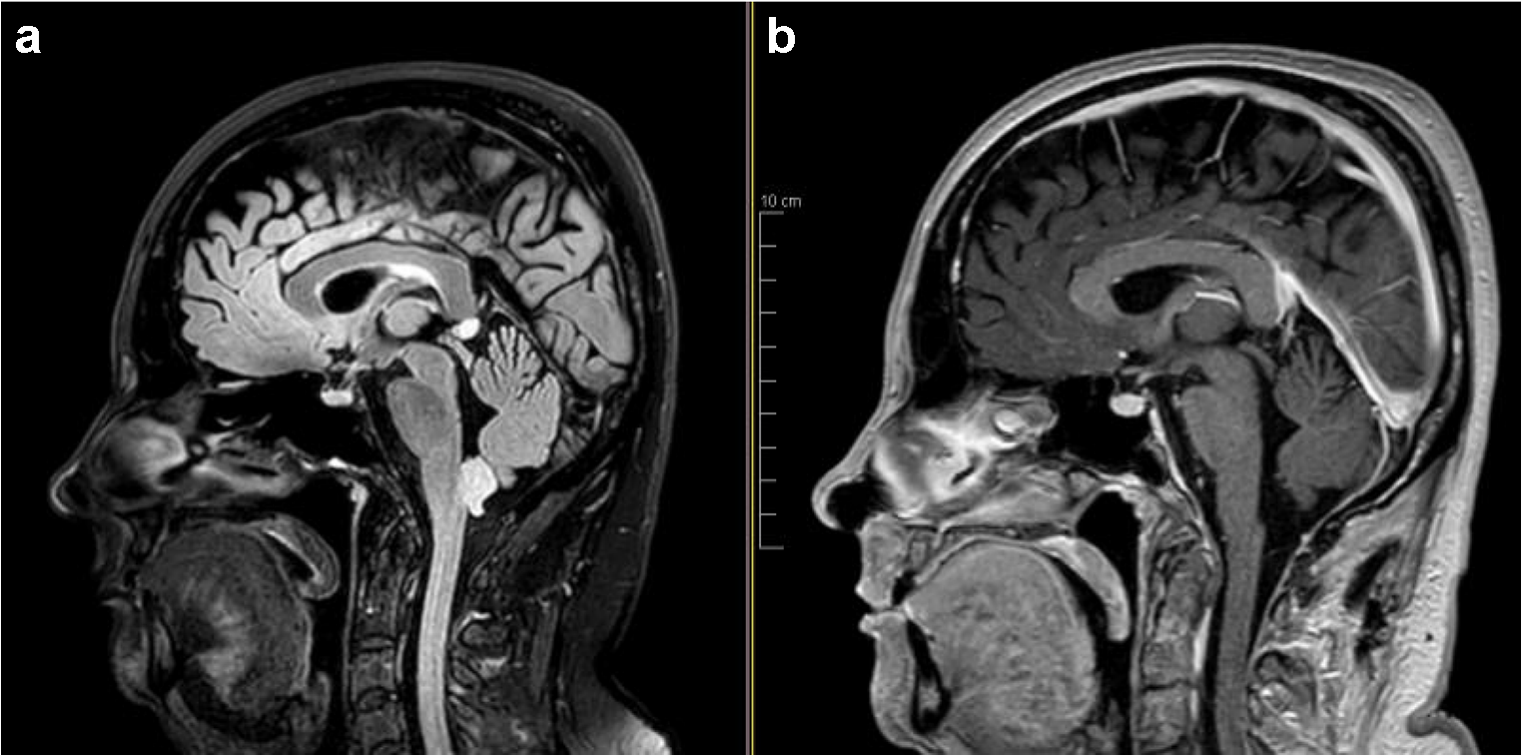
Pre- and postoperative Magnetic Resonance Imaging (a T2 flair; b T1 gadolinium), showing the complete resection of the lesion
